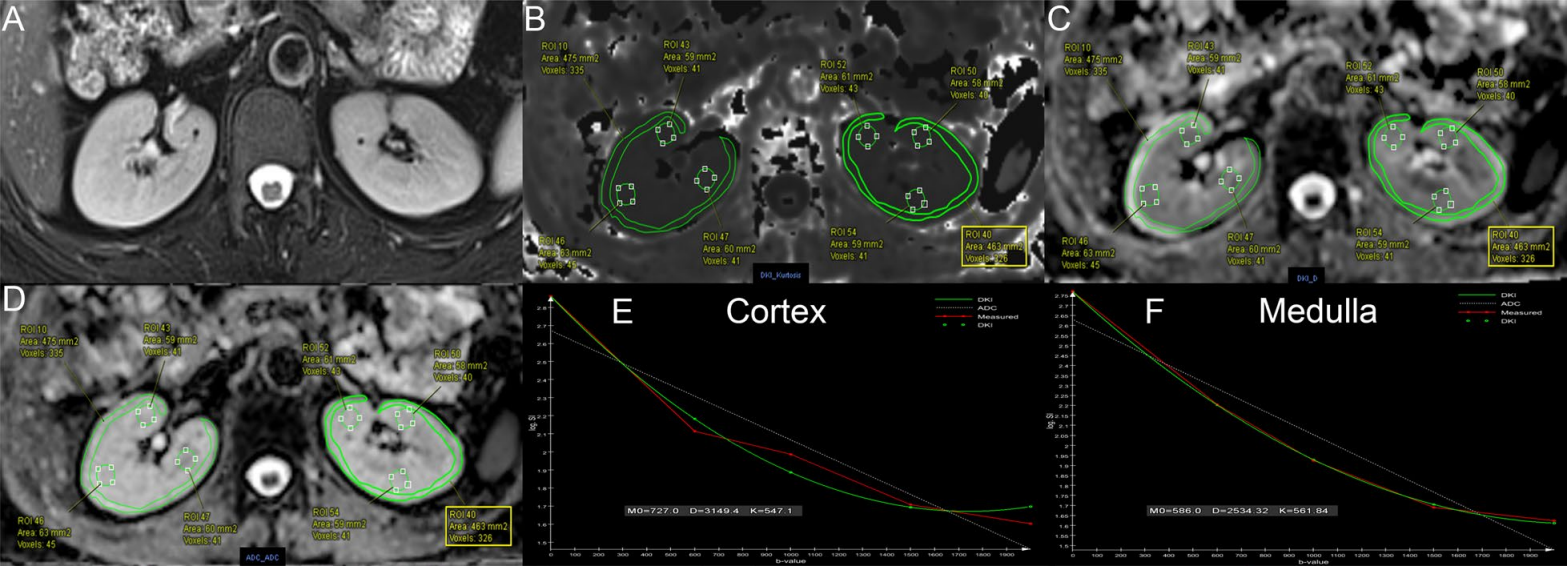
Respective MRI images of healthy volunteers with eGFR = 109.5 mL/min/1.73 m^2^. A–F axial T2-weighted image, MK, MD, ADC, cortex DKI fit, medulla DKI fit, respectively
